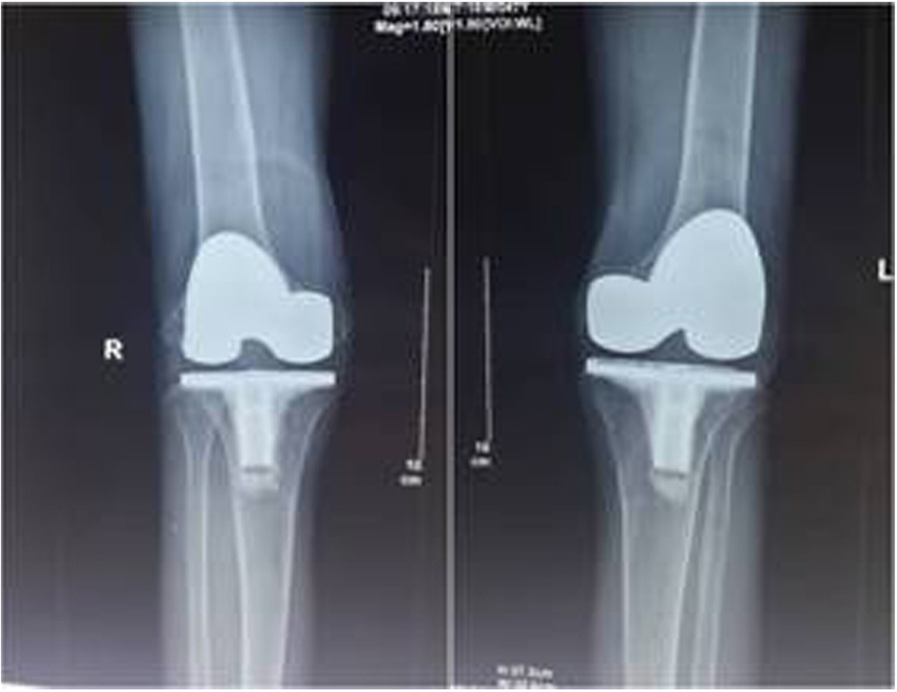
A postoperative anteroposterior radiograph showing satisfactory alignment of the prostheses
